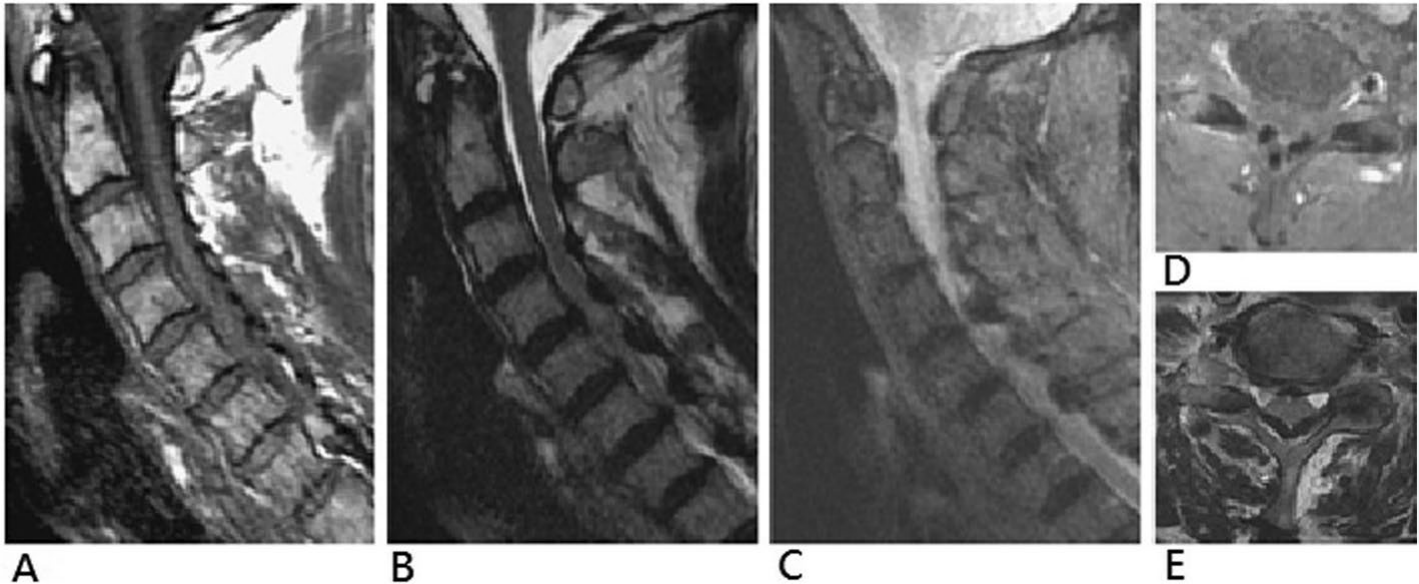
Magnetic resonance imaging of case 1. Nodular masses with low-signal intensity in both T1-weighted imaging (A and D) and T2-weighted imaging (B and E) were observed at the interlaminar spce of C4–5, C5–6, and C6–7. These lesions were surrounded by areas of intermediate-sginal intensity area on T1-weighted imaging and by areas of high-signal intensity area in T2-weighted imaging, and the short tau inversion recovery (STIR) sequence (C) was used to demonstrate the edematous change
